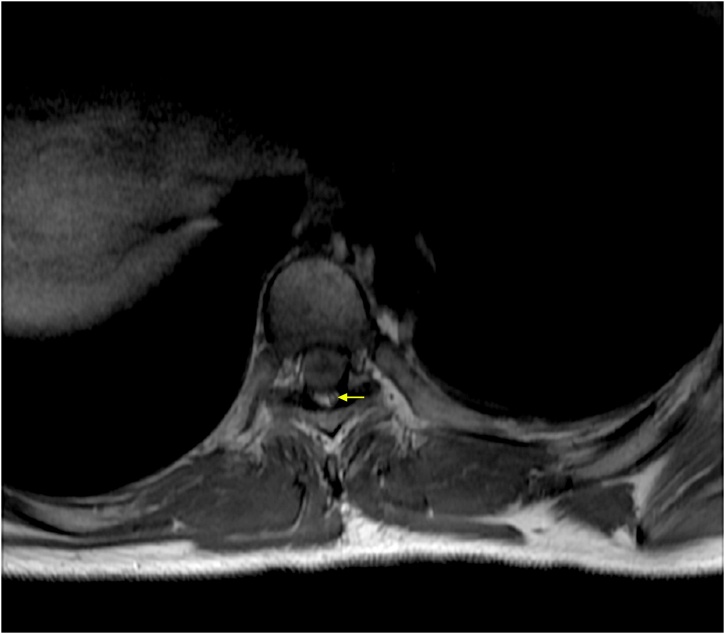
Axial T1-weighted MRI of T6 level, showing an intradural hypointense mass.
